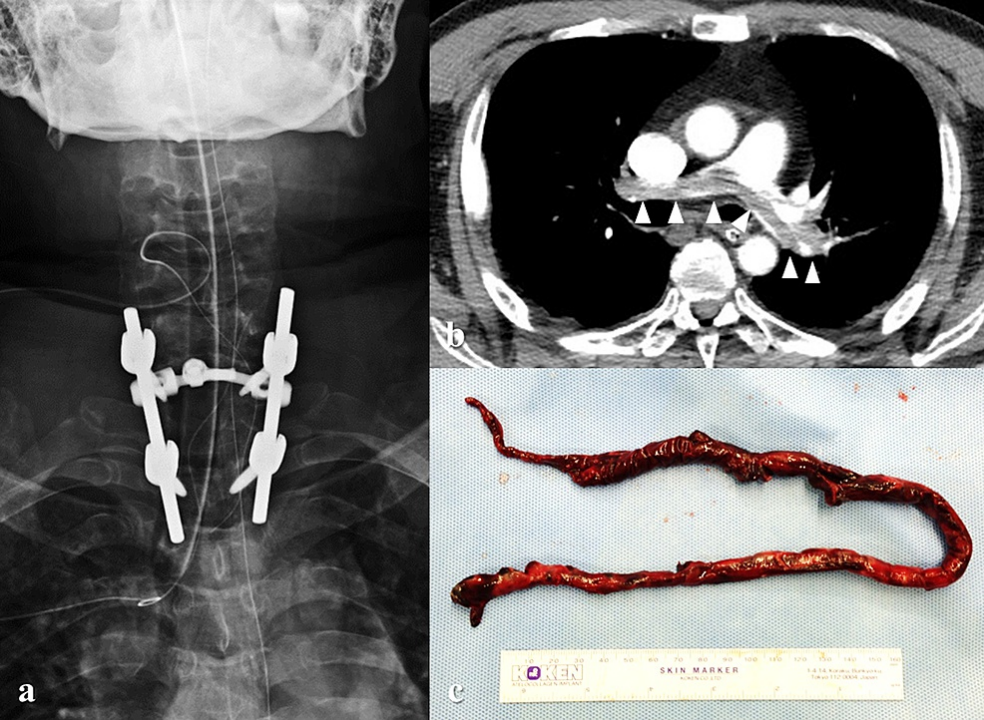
Postoperative images. (a) X-ray taken immediately after spinal surgery. C7-T2 posterior fusion and C7 and T1 laminectomies were performed. (b) Contrast-enhanced CT at three days after surgery. Extensive defect of contrast medium in the area ranging from the main pulmonary artery to the pulmonary artery bifurcation extending into both trunks (arrowheads). (c) The main pulmonary artery was incised to remove a giant thrombus (39 cm).
